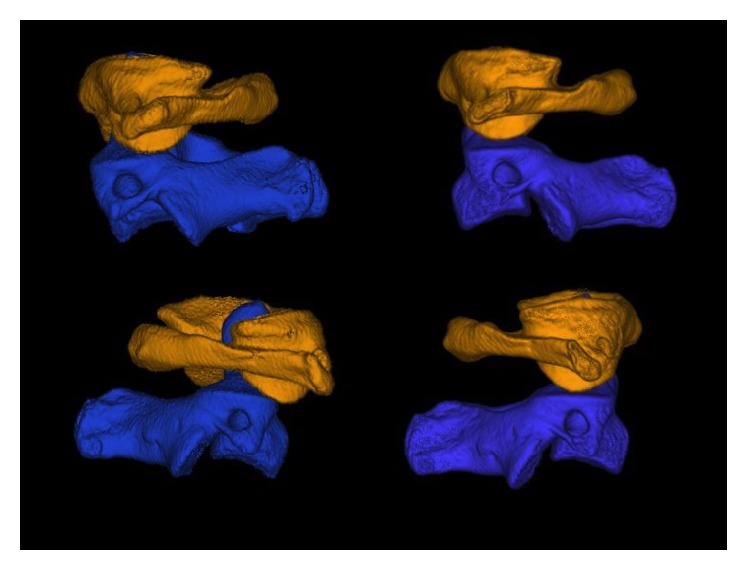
Lateral view from both sides before and after reduction in CT-VRT with segmentation of C1 and C2 (volume rendering technique).
